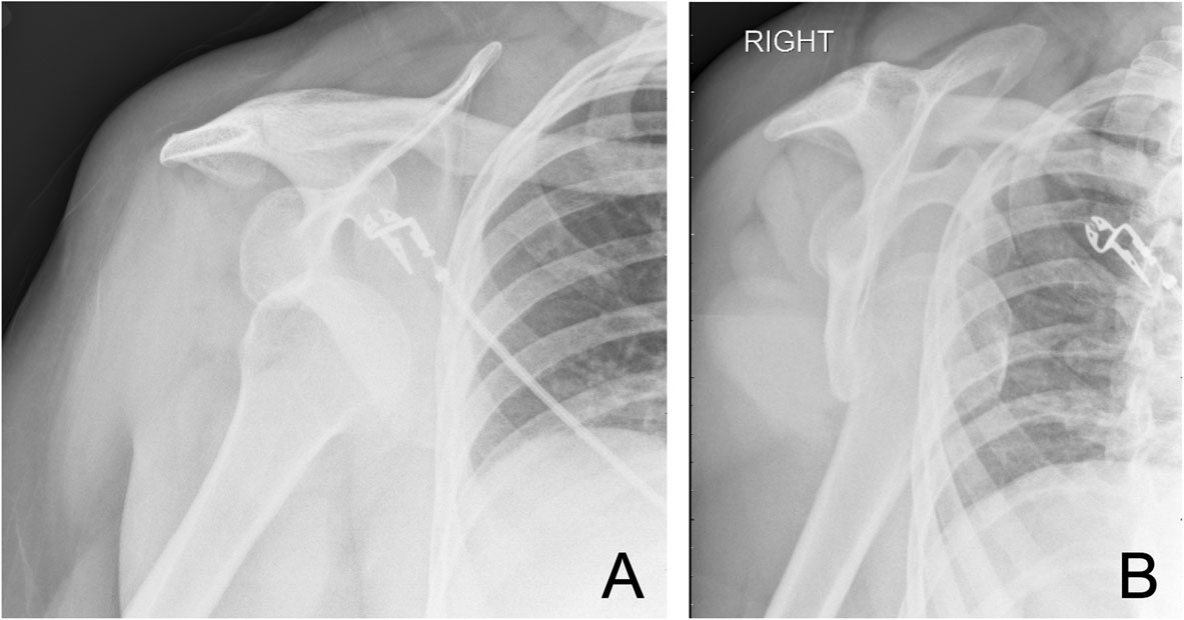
Pre-reduction plain radiographs. a AP view of the right shoulder prior to reduction. b Scapular Y view of the right shoulder prior to reduction
